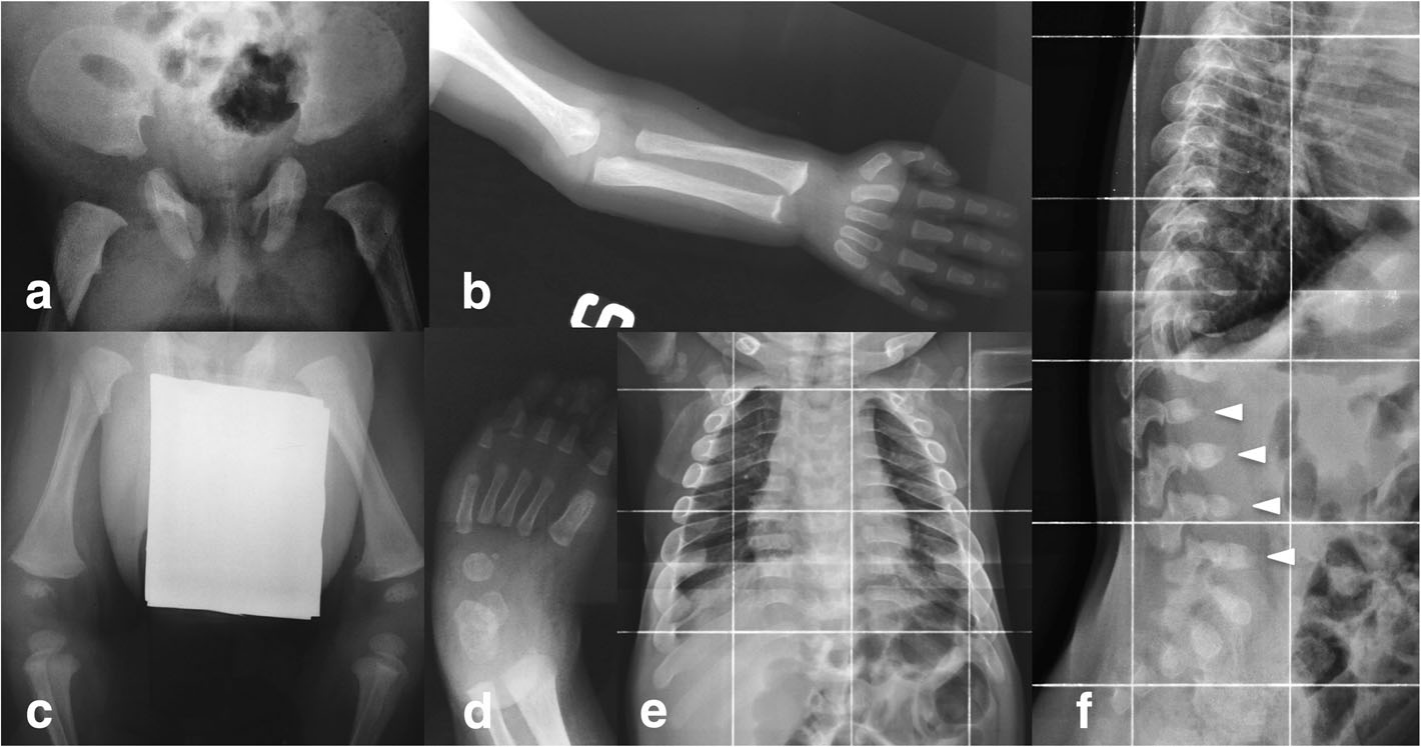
X-rays of an MPS VII infant at the age of 3 months (a–d) and 12 months (e, f) showing generalized skeletal dysplasia (dysostosis multiplex). The pelvis (a) shows typical imaging features characterized by rounded iliac wings and inferior tapering of the ilia with an undeveloped acetabulum; proximal epiphysis of the femurs are not ossified. The femurs (c) are short with hypoplastic epiphyses, similarly to the upper left limb (b) in which cortical thinning, flared metaphysis, and metacarpal widening are also identifiable. The hand (b) and foot (d) are typically dysmorphic: broad and short metacarpals and bullet-shaped phalanges. In the antero-posterior projection of the thorax (e), a marked thickening of the ribs is evident (oar shaped ribs). In the latero-lateral projection of the dorsal and lumbar spine (f), the lumbar vertebral bodies from L2 to L5 are markedly wedge deformed with anterior beaking aspect (arrows), and with angulation of the dorsal-lumbar tract
